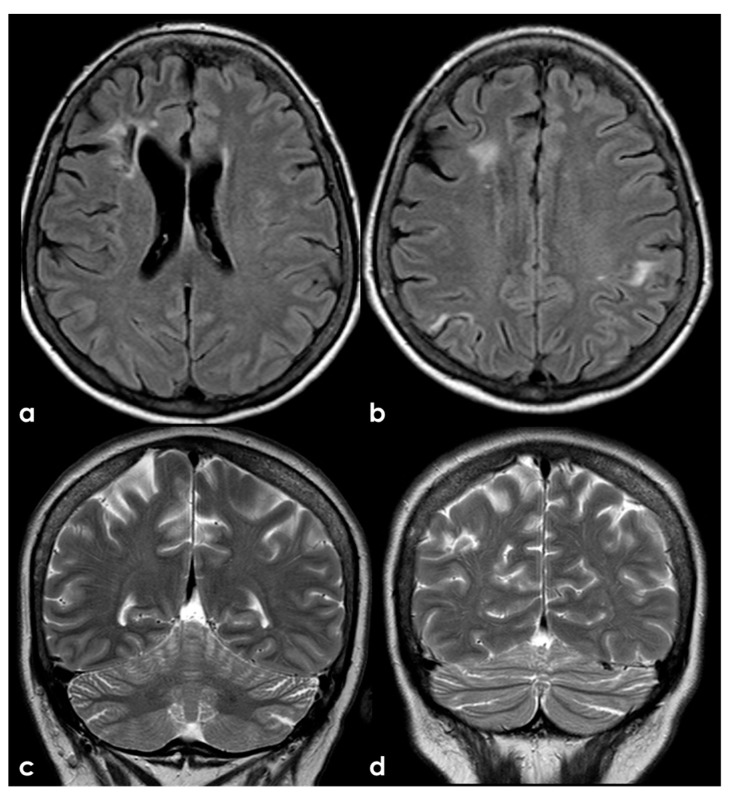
Brain MRI (axial FLAIR in panels (a,b) and coronal T2W sequence in panels (c,d)) showing multiple cortico-subcortical ischemic lesions on both hemispheres and only few mildest SVD markers.
